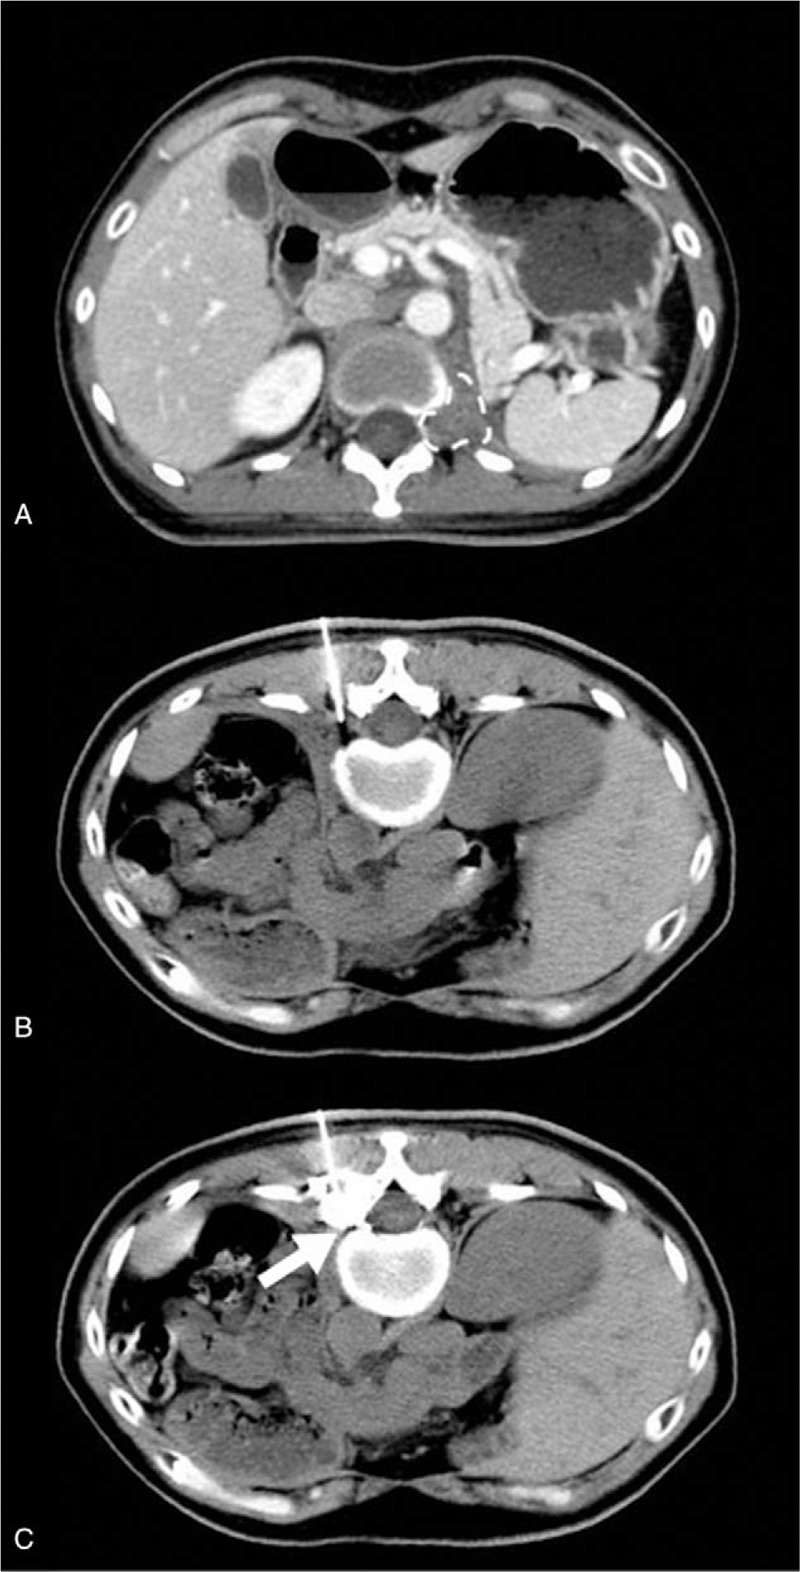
Computed tomography (CT) guided paravertebral doxorubicin injection: (A) CT image revealing the metastasis in the vertebral and intervertebral T12 foramina (dotted line area); (B) the inserting target was located near the left intervertebral foramen of T12-L1 level; (C) CT image confirming the contrast agent diffused through the intervertebral foramen into the dorsal epidural space.
